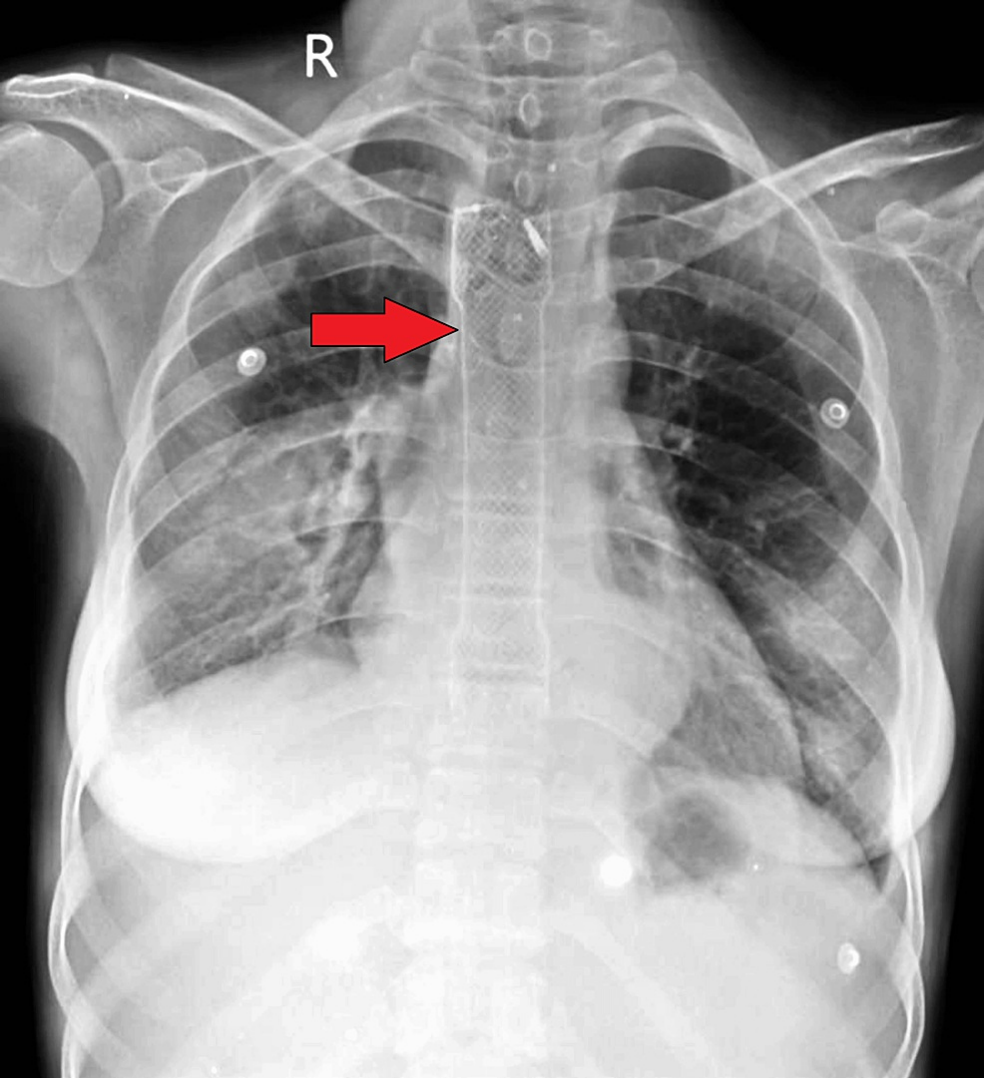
A chest X-ray posteroanterior view, showing the placement of the esophageal stent
